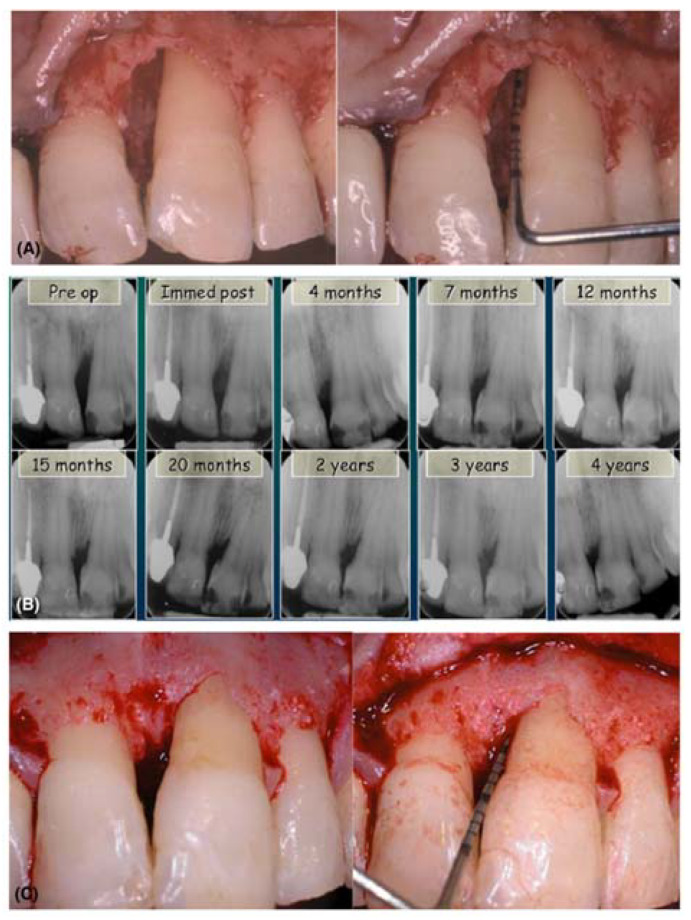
(A) Pre-operative clinical images showing a deep intrabony defect, (B) X-rays depicting appreciable defect fill following treatment with EMD in combination with a bone graft, and (C) four year outcome following intrabony defect repairment with EMD. Reprinted from [140] with permission from Wiley Online.
